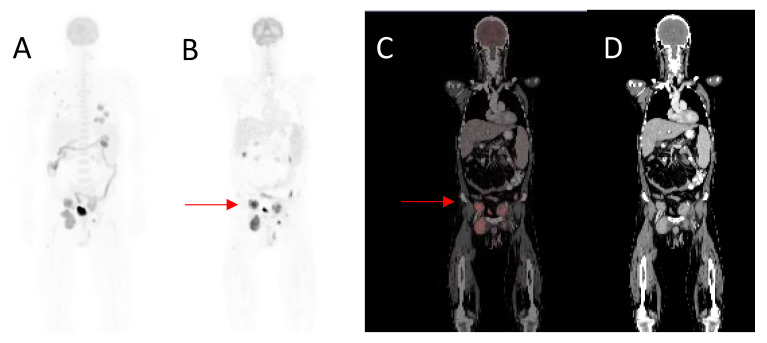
A 50-year-old male, diagnosed with malignant melanoma of the right groin with inguinal lymph node metastases, excision and resection of metastatic inguinal nodes, presented with right groin recurrence. Maximal intensity projection image (A), coronal ^18^F-FDG- PET (B), fused (C) and CT (D) images demonstrating right inguinal recurrence (arrow). He also had abdominal and retroperitoneal lesions. MTV 174.59 cm^3^, TLG 1611.46, SUVmax 22.12, whole-body MTV 780.55 cm^3^ and whole-body TLG 28,279.33. Overall survival was 5 months.
